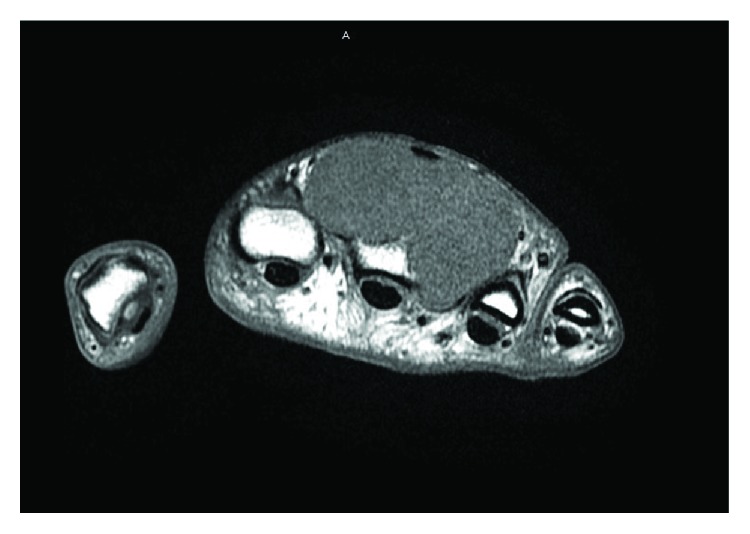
T1 axial MRI image of the right hand demonstrating an expansile destructive mass involving the proximal phalanx of the third digit measuring 3.8 × 2.2 × 3.5 cm.
